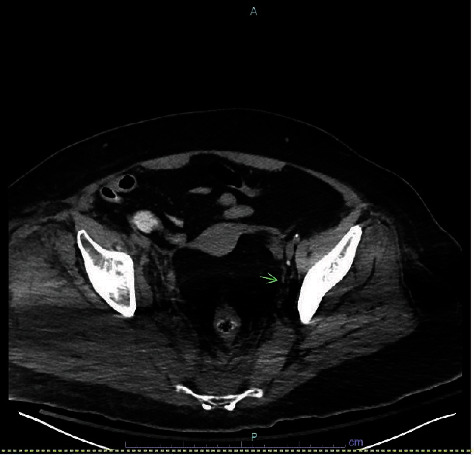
CT pelvis demonstrating asymmetrical swelling and stranding affecting the right piriformis muscle with an arrow pointing to ureteral involvement in muscle stranding.
